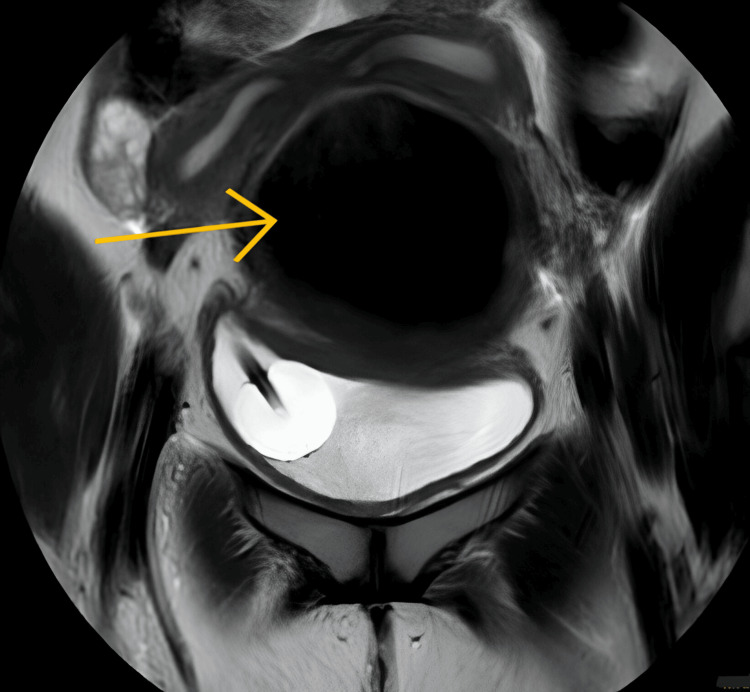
MRI showing hematocolpos and well-distended bladder with a Foley bulb in situ MRI: magnetic resonance imaging
